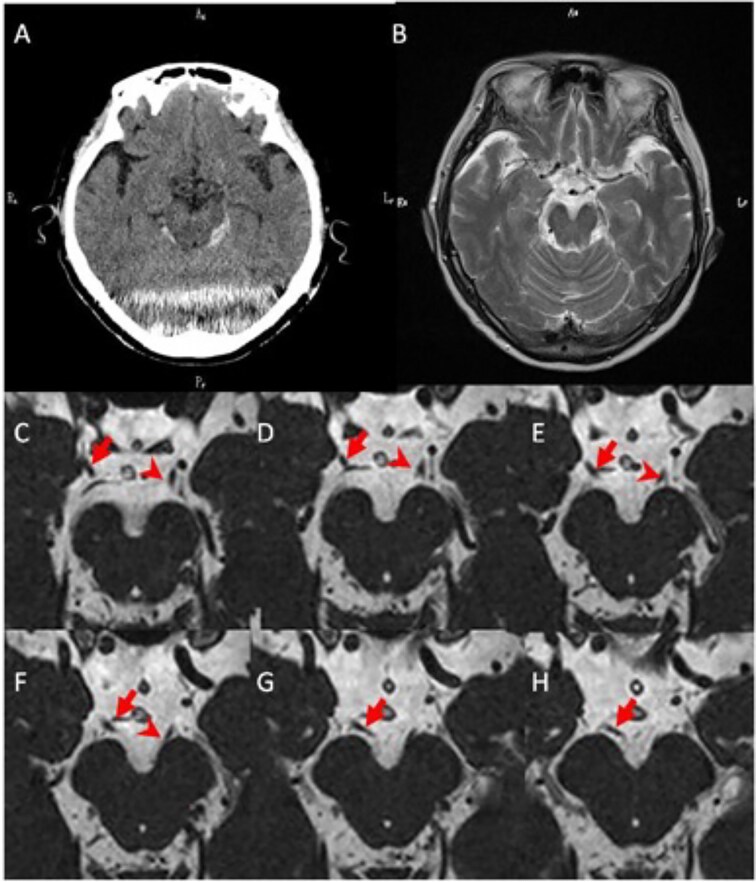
Brain CT on admission and MRI on day 3. (A) A head CT scan showing SAH findings in the bilateral ambient cisterns. (B) MRI T2 images showing no intraparenchymal brain damage. (C–H) Images C–H are MRI CISS images from anterior to posterior. The distal end of the right oculomotor nerve is forming a blind stump (arrow). The left oculomotor nerve is normally connected to the midbrain (arrowhead). CT, computed tomography; MRI, magnetic resonance imaging; SAH, subarachnoid hemorrhage; CISS, constructive interference in steady state.
